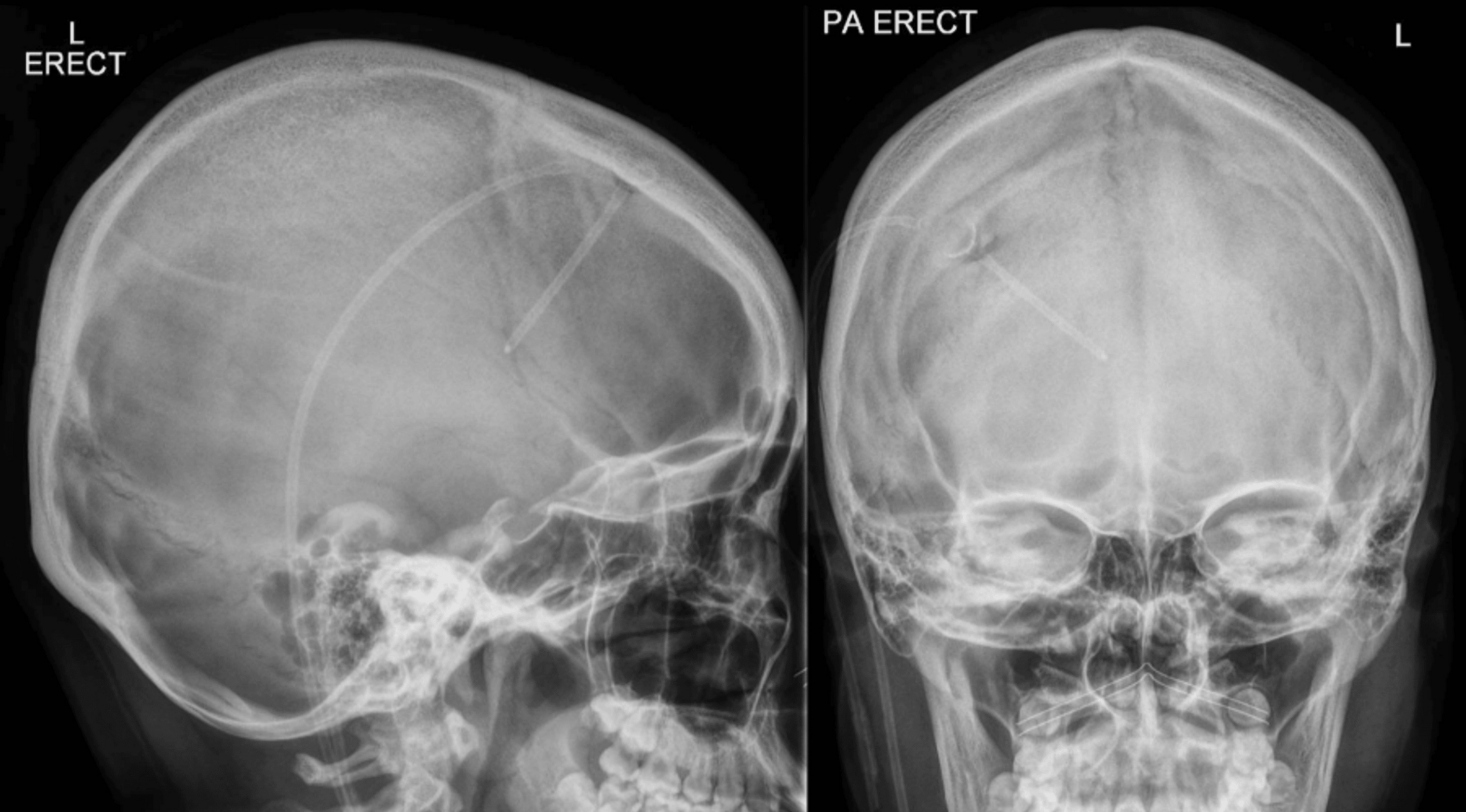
X-ray skull imaging in anteroposterior and lateral views.
